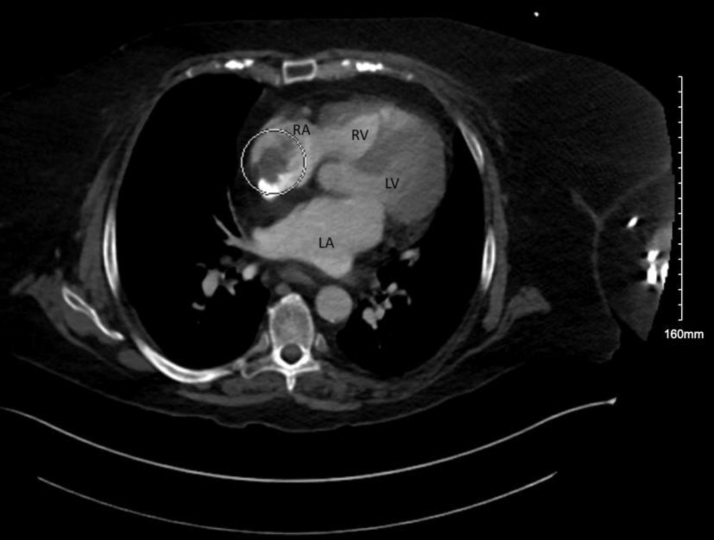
Computed Tomography Showing a Right Atrial Mass LA = left atrium; LV = left ventricle; RA = right atrium; SVC = superior vena cava.
